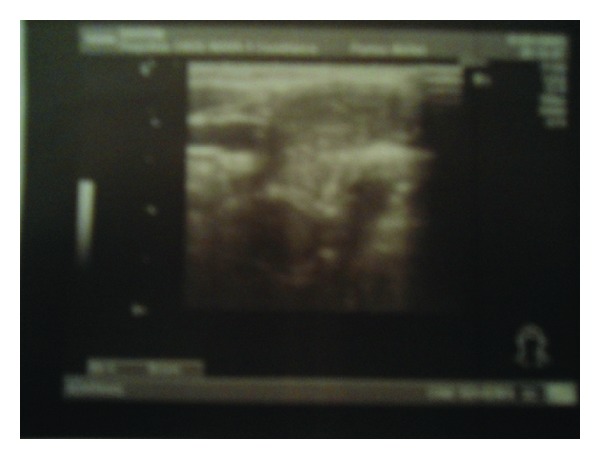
High resolution ultrasonography: right thyroid lobe having a hypoechoic, heterogenous.
